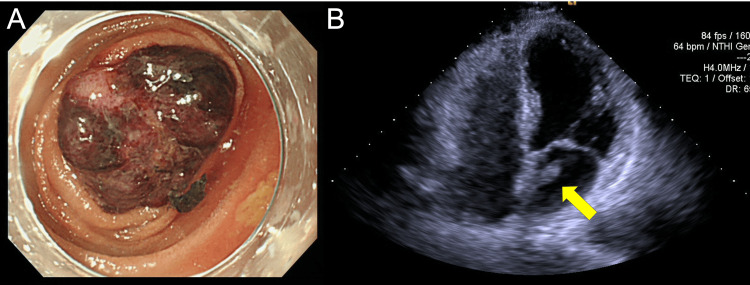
(A) Preoperative enteroscopy showing a 30-mm elevated lesion in the jejunum. (B) Preoperative transthoracic echocardiography revealing a mobile mass in the left atrium (arrow).
